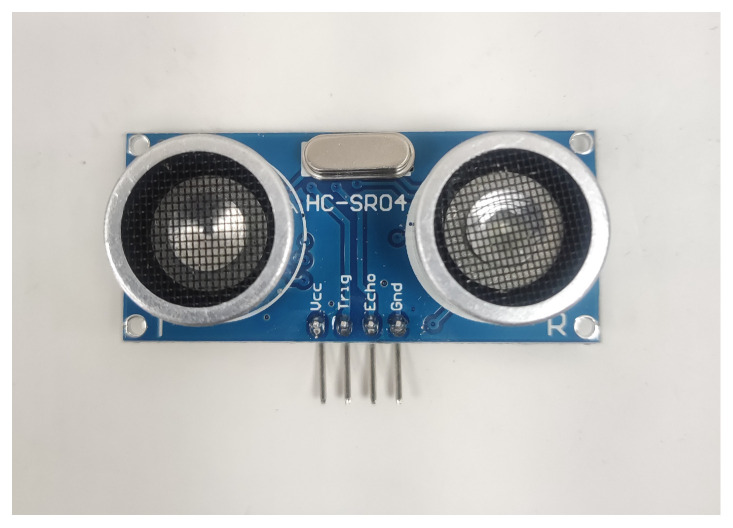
HC-SR04 ultrasonic sensor.
